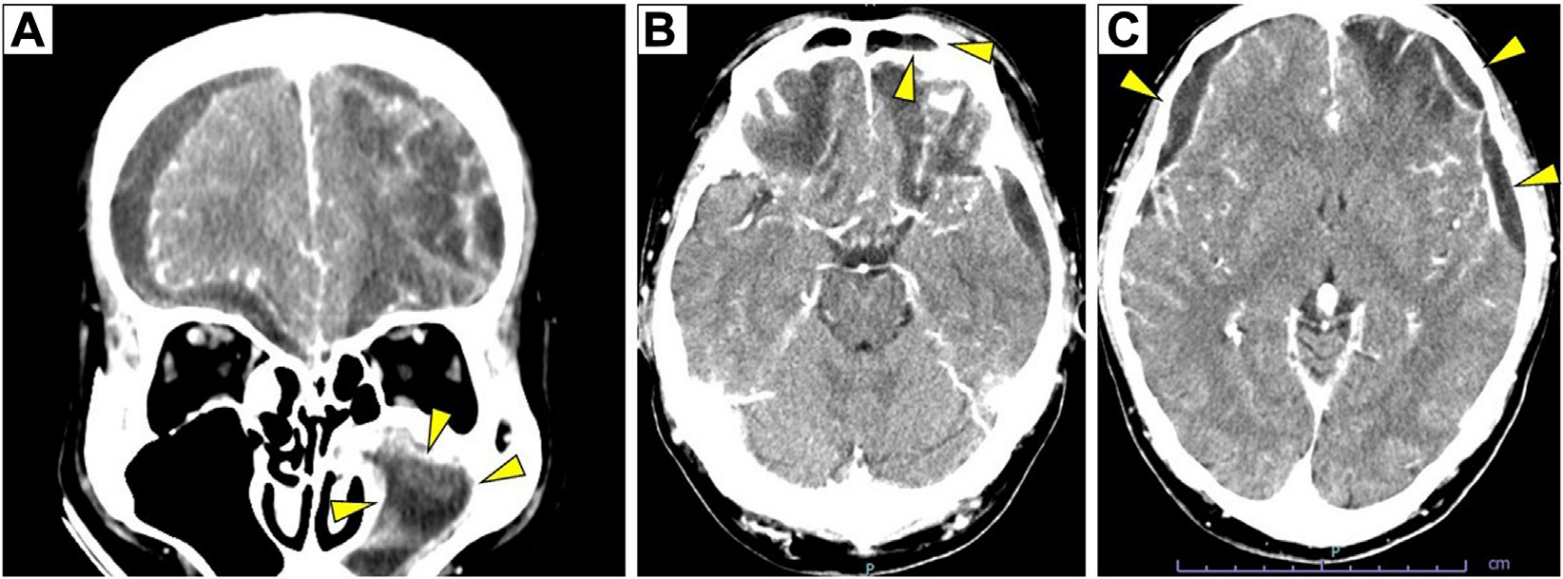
Contrast-enhanced computed tomography of the head. Contrast-enhanced computed tomography imaging demonstrates left-sided maxillary and frontal sinusitis (A, B), with evidence of multiple subdural abscess formations (C).
